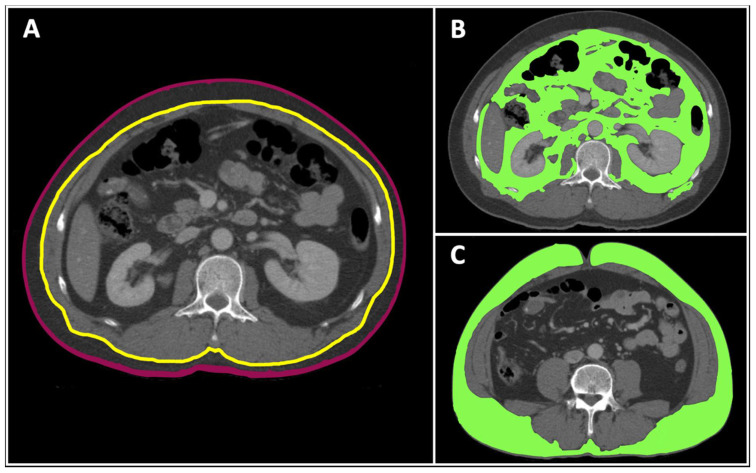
This figure demonstrates the morphomic variable measurements of a 53-year-old male motorcyclist by a CT scan. Total body area was measured by the cross-sectional area inside the purple line (A), whereas the visceral fat area (at the L2 vertebral level) was represented by the area inside the fascia (yellow line) meeting fat density thresholds (B), and the subcutaneous fat area (at the L4 vertebral level) was represented by the area between the skin and fascia meeting fat density thresholds (C).
